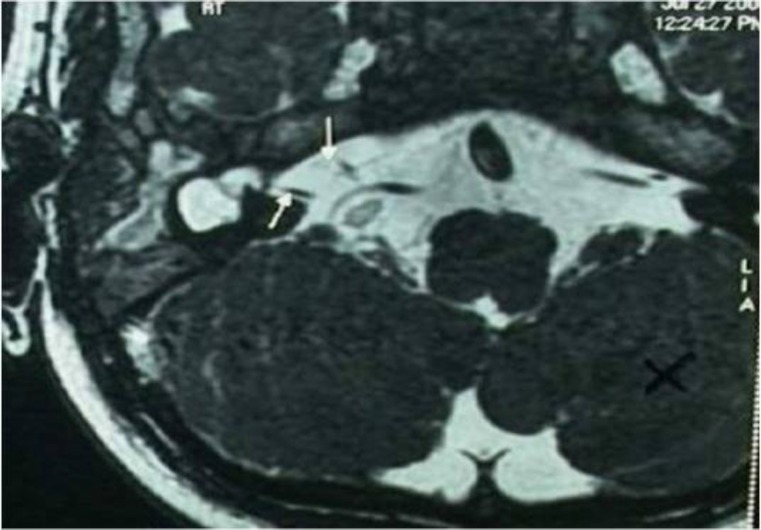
MRI brain showing an absent vestibulo-cochlear nerve complex on the left side and partial visualization on the right side
